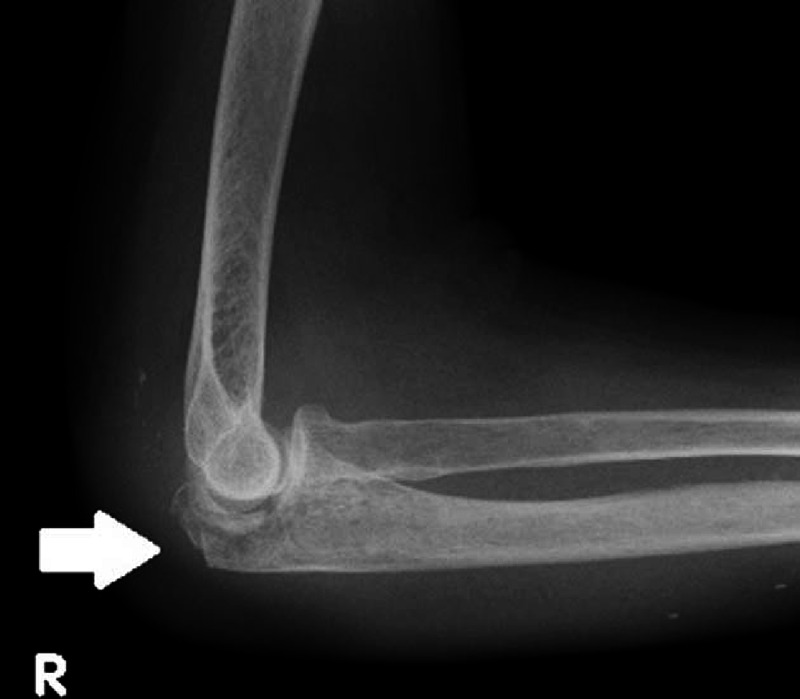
X-ray of the patient's right elbow joint. Osteolysis is seen (arrow).
